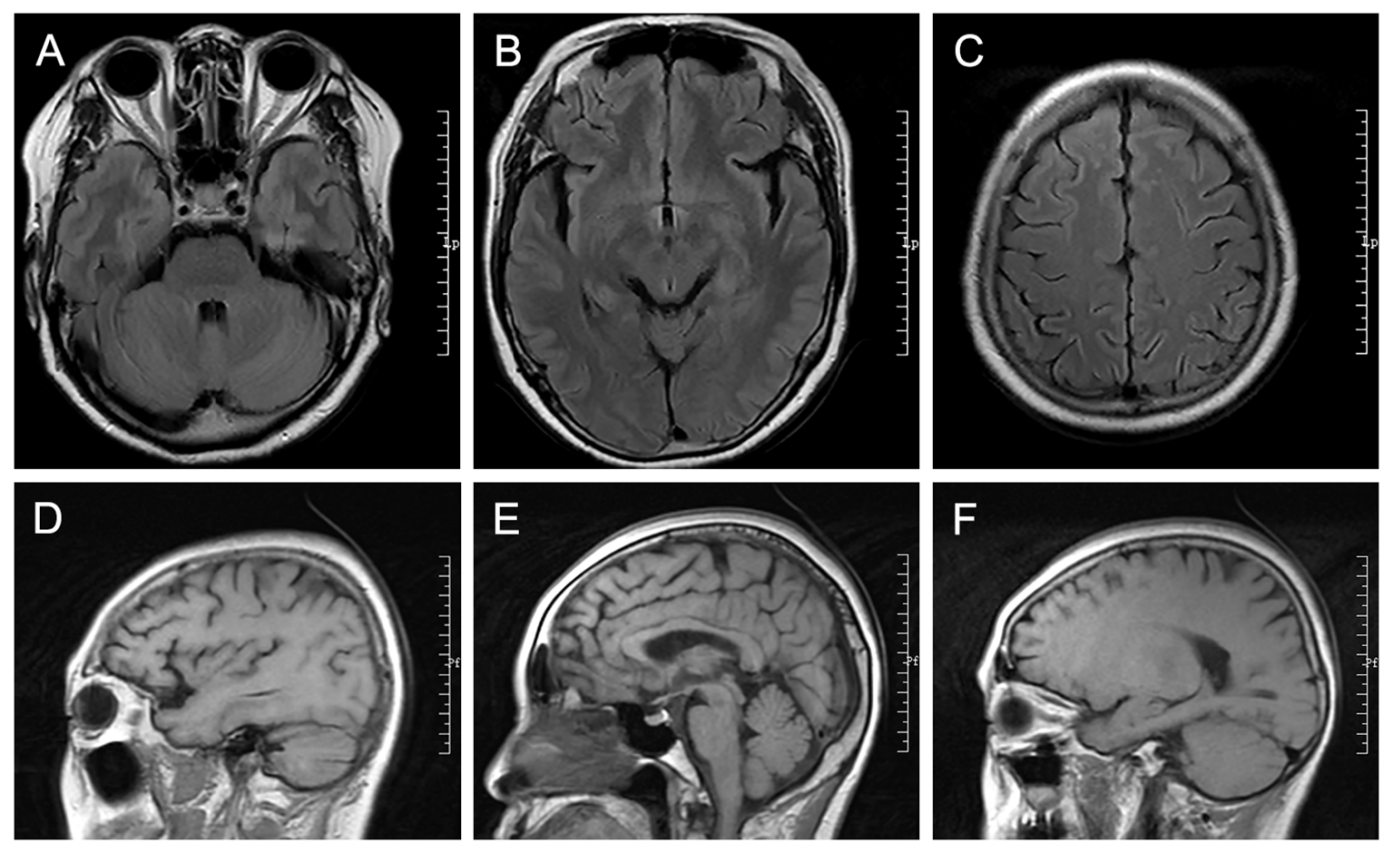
The radiology images. (A-C) The cross-sectional T2 FLAIR MRI. (D-F) The sagittal T1 FLAIR MRI.
